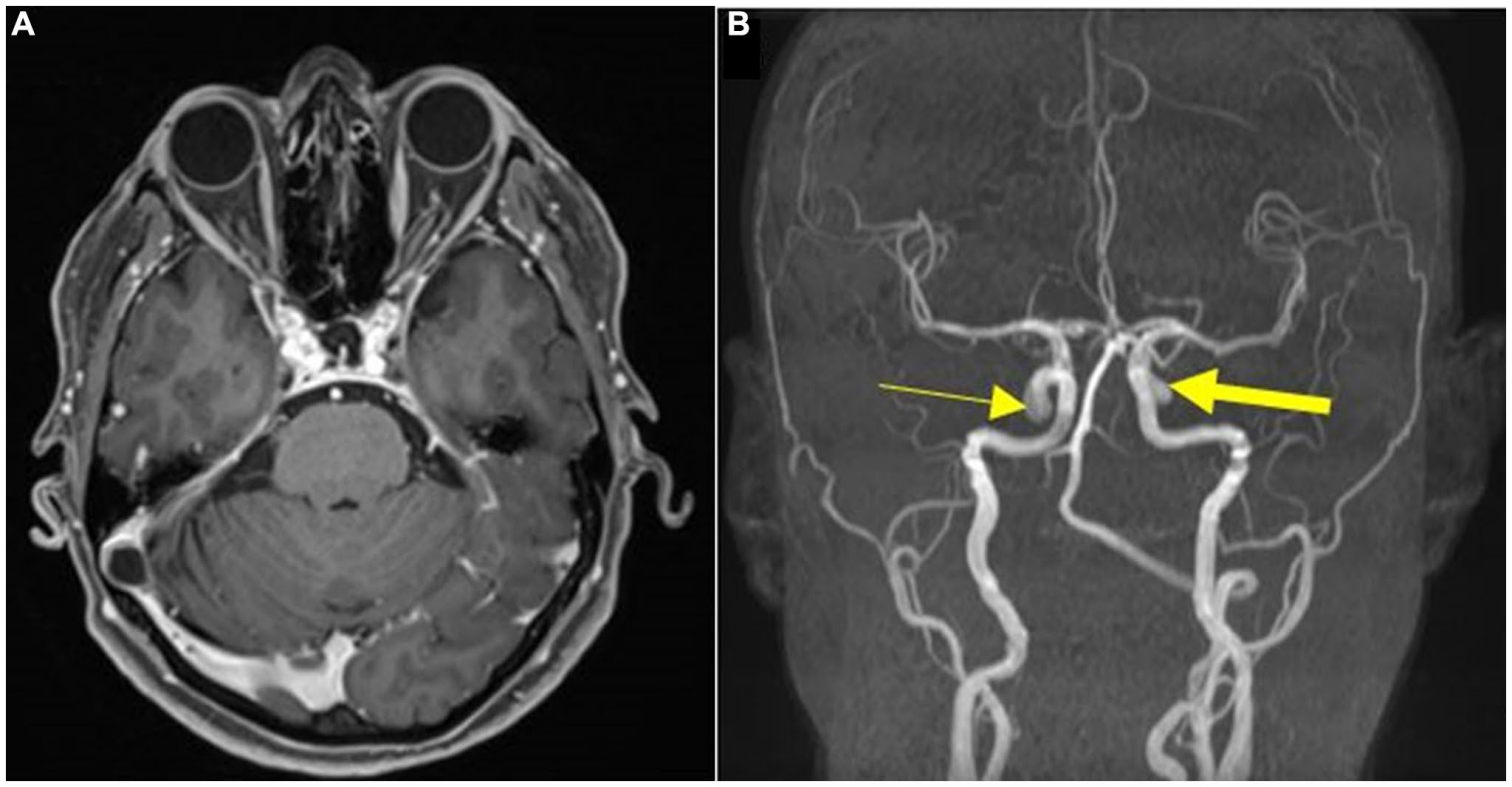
(A) Preoperative post gadolinium MRI T1w axial showing paraclinoid mirror aneurysms. (B) MRA revealing a right-sided paraclinoid aneurysm (thin arrow) and a left-sided paraclinoid aneurysm (thick arrow).
